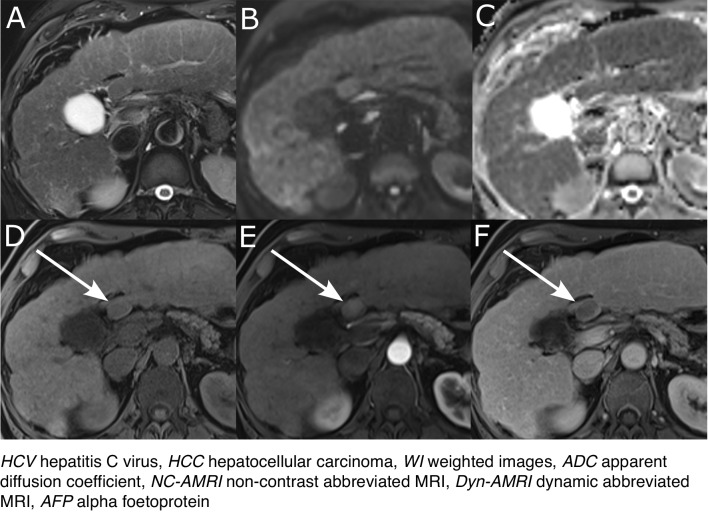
HCC patient false negative on NC-AMRI and true positive on Dyn-AMRI and NC-AMRI + AFP. A 57-year-old male patient with HCV cirrhosis and HCC. Abbreviated MRI shows a 20-mm nodule in segment IV, which is isointense on T2WI (A), without restricted diffusion and corresponding ADC map (B and C respectively). The same nodule is isointense on unenhanced T1WI (D, arrow) with homogenous arterial enhancement on arterial phase image (E, arrow) and washout and capsule on portal venous phase (E, arrow). NC-AMRI was therefore scored as negative, while Dyn-AMRI and complete MRI were scored as LI-RADS 5 (positive). NC-AMRI + AFP was considered positive, with an AFP value of 9.5 kUI/l
